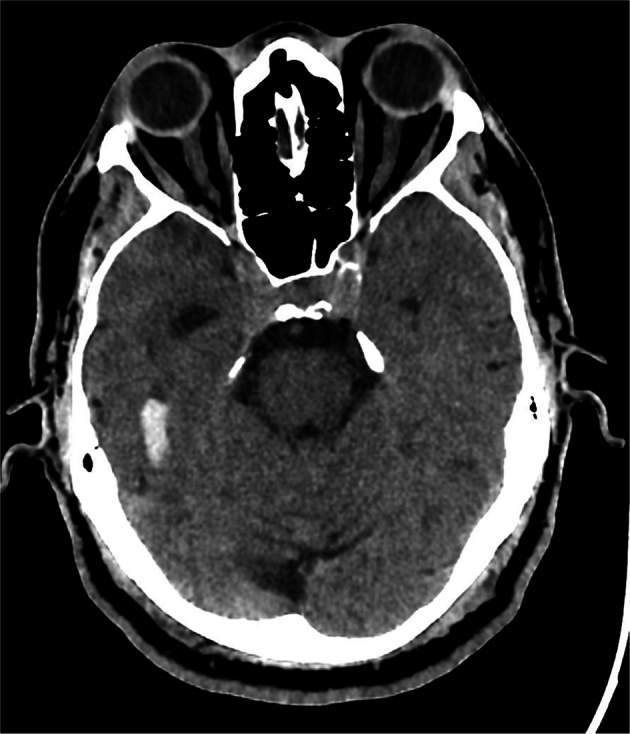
CT image of the head with a right temporal parenchymal hemorrhage. GPT-4V correctly identified the image modality, anatomical region, and pathology of “Intracranial hemorrhage”
